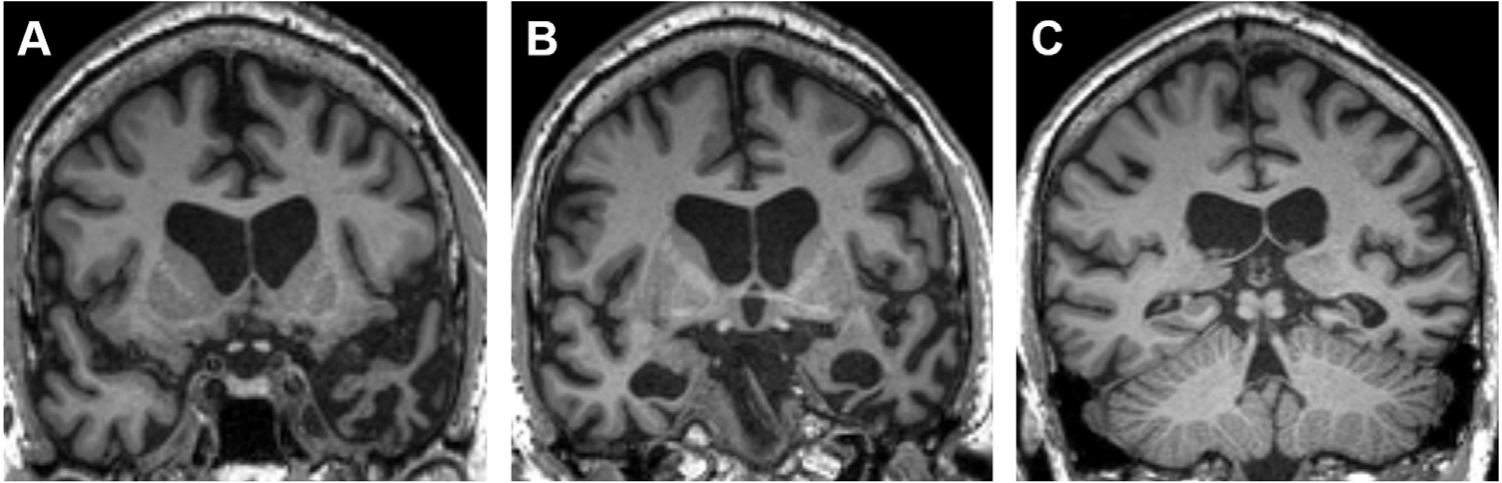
Brain MRI findings in patient BA. Coronal sections of BA's T1-weighted volumetric brain MRI through the temporal poles (A), mid-anterior temporal lobes (B) and temporo-parietal junctional zones (C) show asymmetric, focal temporal lobe atrophy typical of semantic dementia. There is more severe involvement of the left temporal lobe (projected here on the right) and within each temporal lobe, marked atrophy of the pole, inferior temporal cortex and mesial temporal structures, with relative sparing of superior temporal gyrus and more posterior cortices.
